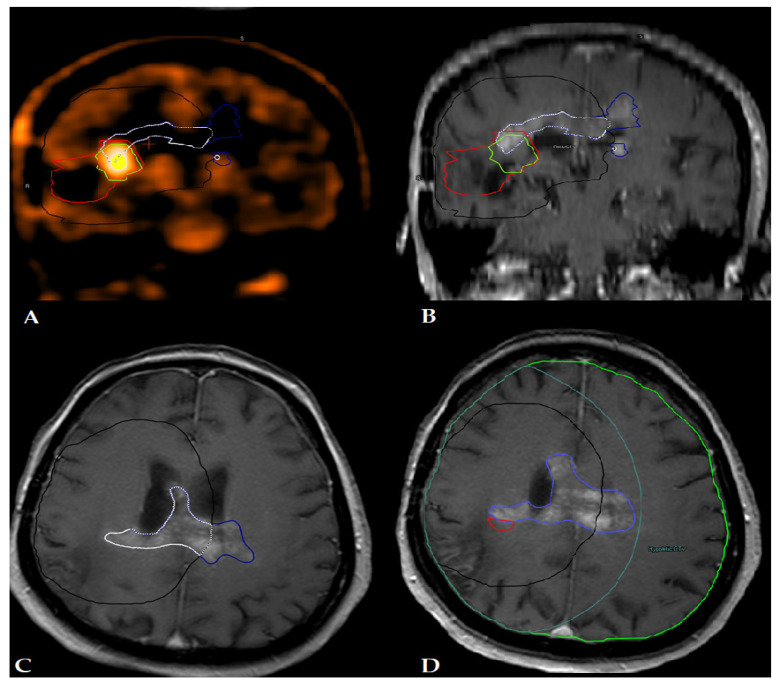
Patient number 2 shows post-contrast T1-weighted MRI (A,C,D) and 11C-MET PET (B) brain images. CTV (black), PET-MR GTV (red), and BTV (green) recurrent tumor within CTV (white), recurrent tumor outside CTV (blue), and hypothetic CTV (turquoise blue).
